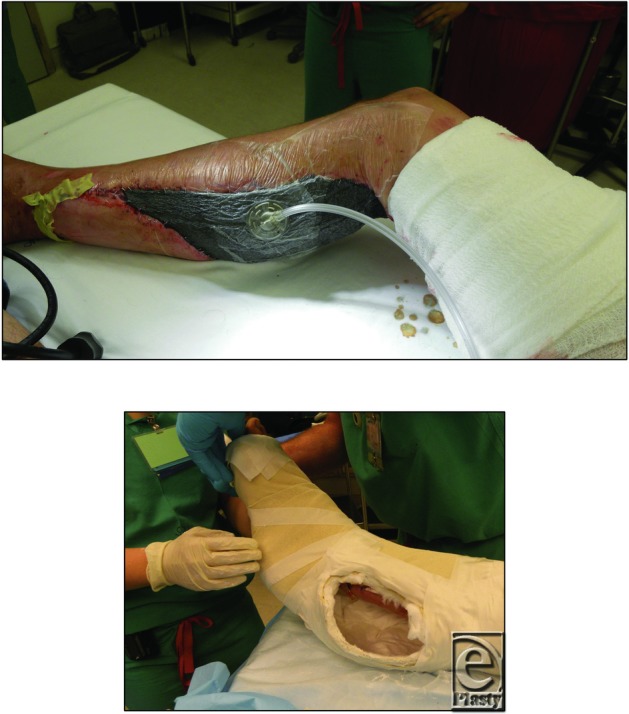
Postoperative care: The skin graft was placed under negative pressure and the flap was monitored with internal Doppler. The right leg was placed in a cast for 5 days with a window to monitor the free flap.
